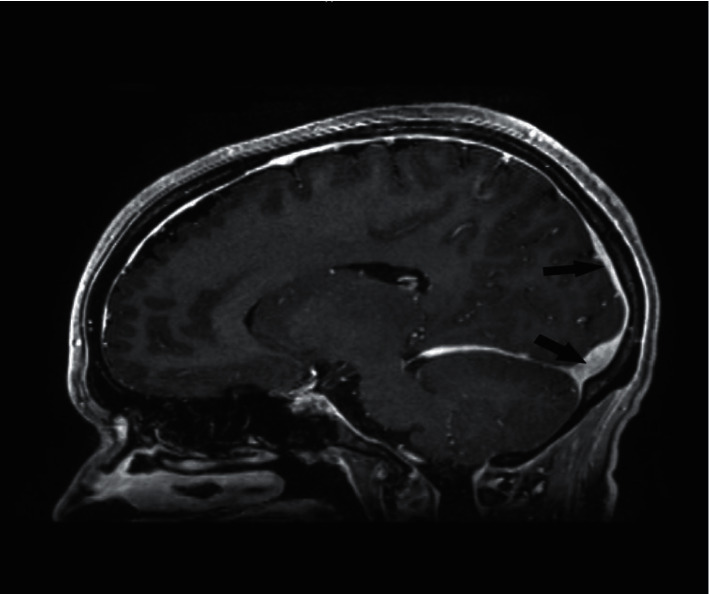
Magnetic resonance imaging (MRI) of the brain with contrast, postcontrast sagittal view showing marked improvement in the previously seen filling defect in the superior sagittal sinus (arrows).
